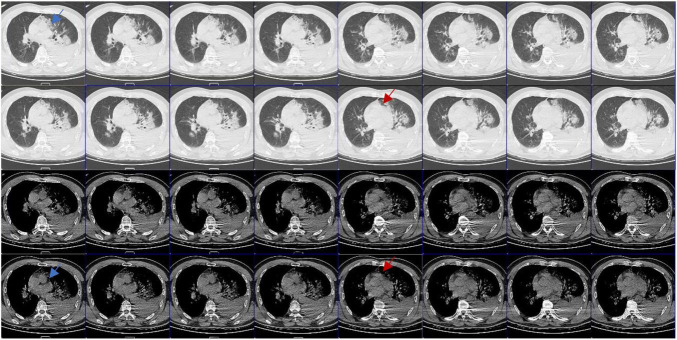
Computed tomography (CT) images in different window settings: As the blue arrow shows, there is no obvious pericardial structure in the left area of pulmonary artery, which is closely related to lung tissue and has irregular edge; as the red arrow shows, there is an abnormal notch near the pulmonary artery, which is rare when the pericardium is intact.
